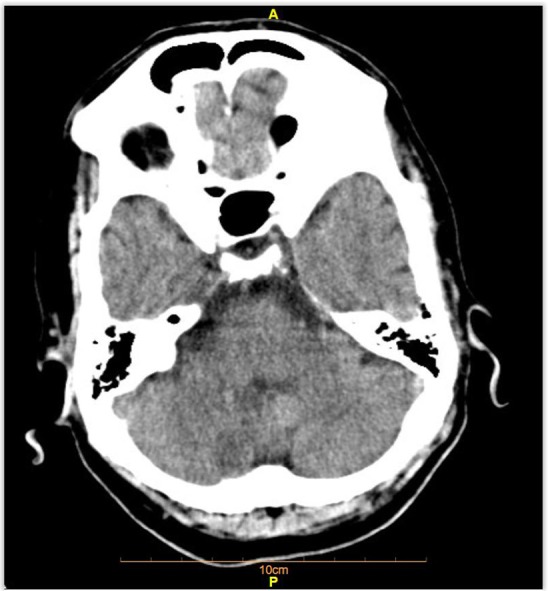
Axial Brain CT scan of the patient Suspicious hypo density focus in posterior inferior aspect of right cerebellar hemisphere
